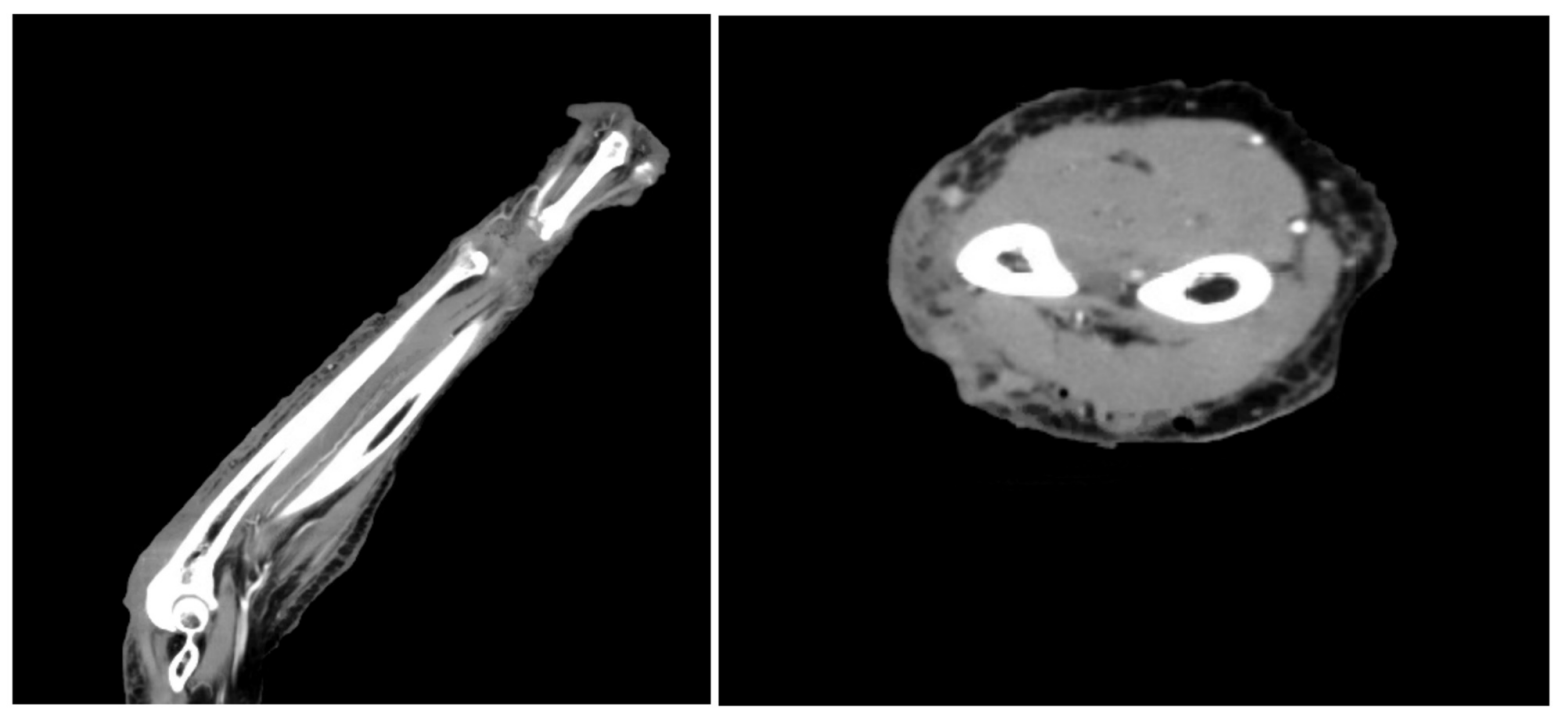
Post-operative CT scans showing no measurable fluid collection
